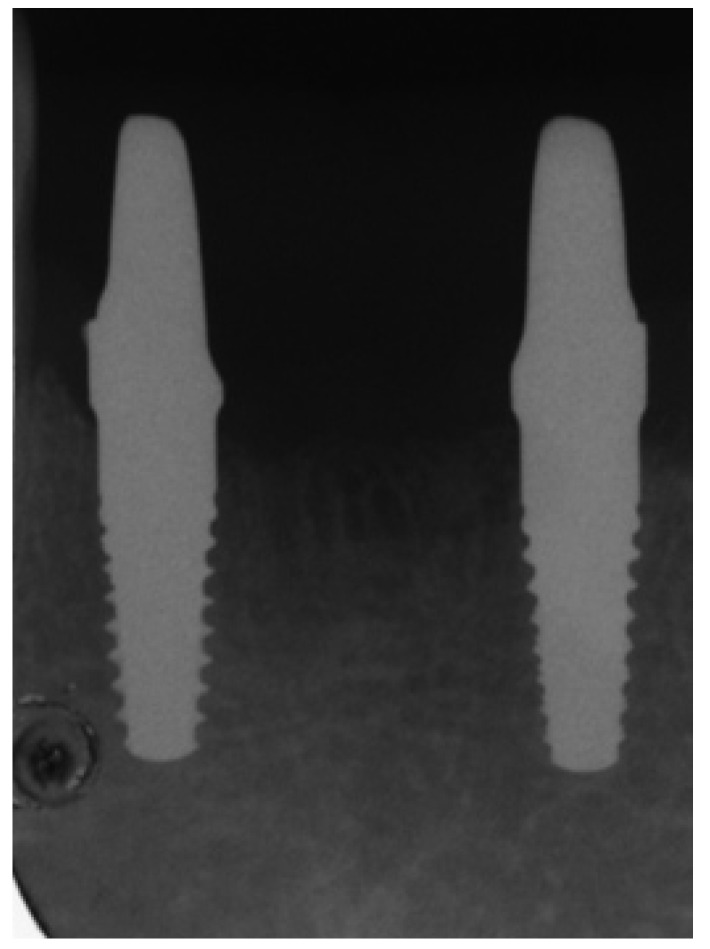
Radiographic control 6 months after implant insertion.
